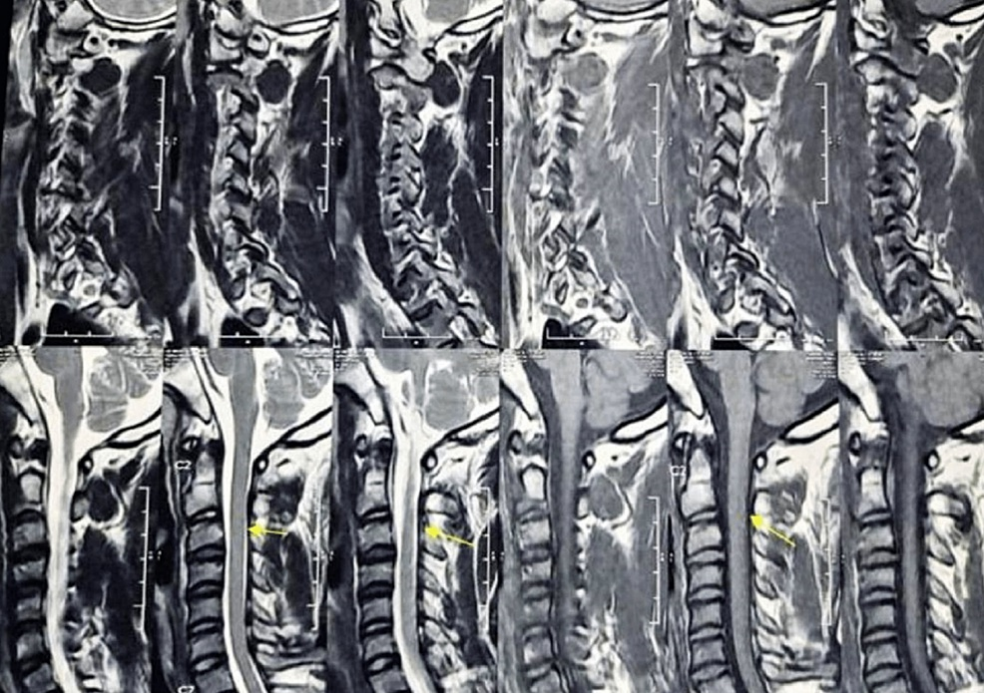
Normal appearing MRI sequence of the cervical region with a mild extension of the neck.
